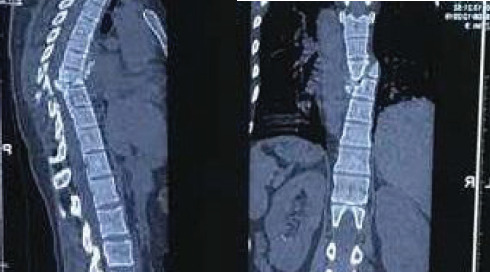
Computed tomography scan of the spine (coronal and sagittal section) showing fracture at T8-T9 level.
